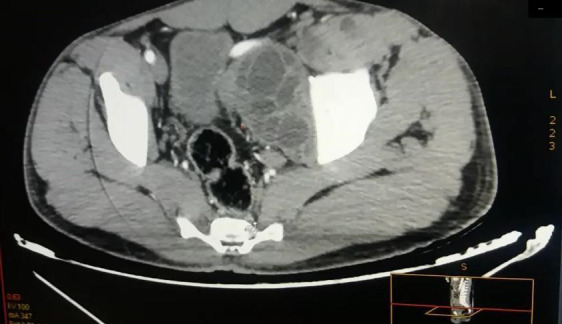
Coupe transversale de tomodensitométrie injectée abdomino-pelvienne montrant une énorme masse au dépend du muscle psoas refoulant la vessie Cross-section of injected abdominal-pelvic CT scan showing a huge mass at the psoas muscle pushing back the bladder
